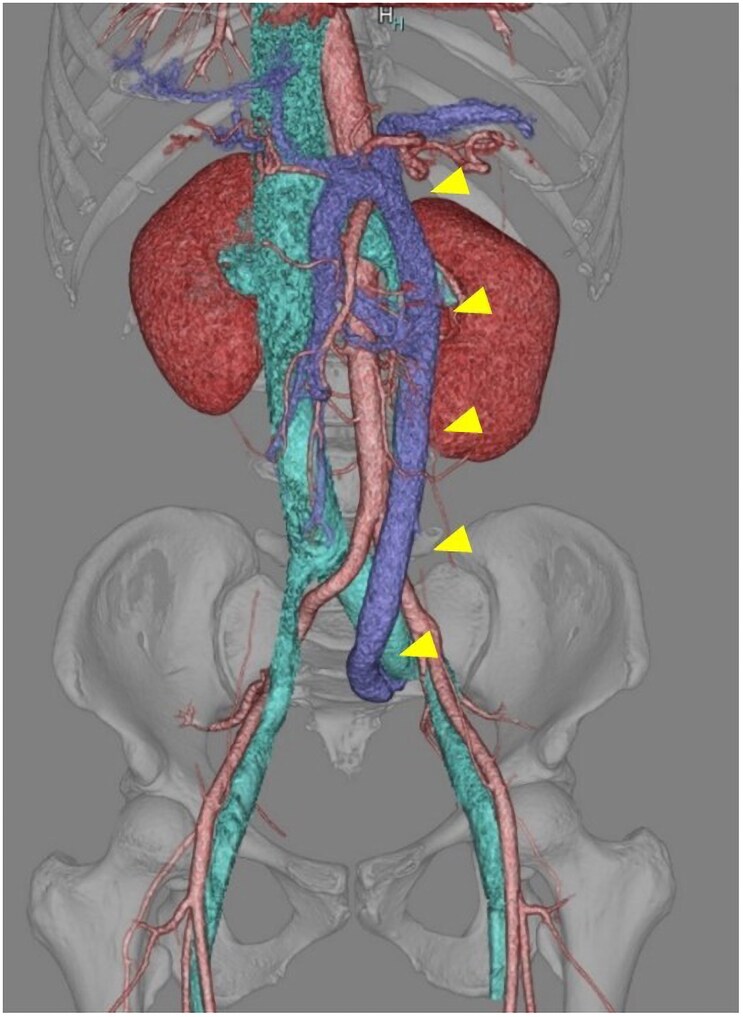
3D reconstructed abdominal contrast-enhanced computed tomography. The congenital portosystemic shunt (CPSS; arrowheads) descends from the main portal vein trunk and flows into the left common iliac vein.
